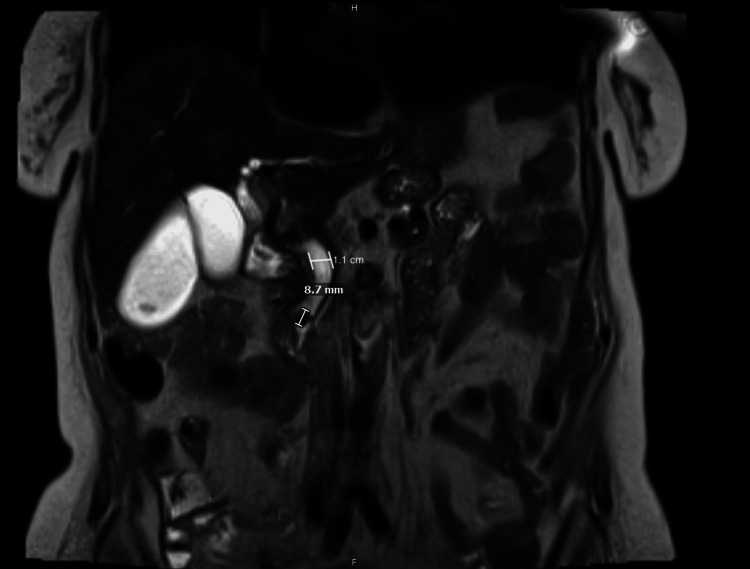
T2-weighted MRCP showing dilation of the intrahepatic and extrahepatic biliary system, with the common bile duct measuring 1.1 cm, and evidence of a distal CBD obstructing stone measuring 8.7 mm MRCP: magnetic resonance cholangiopancreatography; CBD: common bile duct
